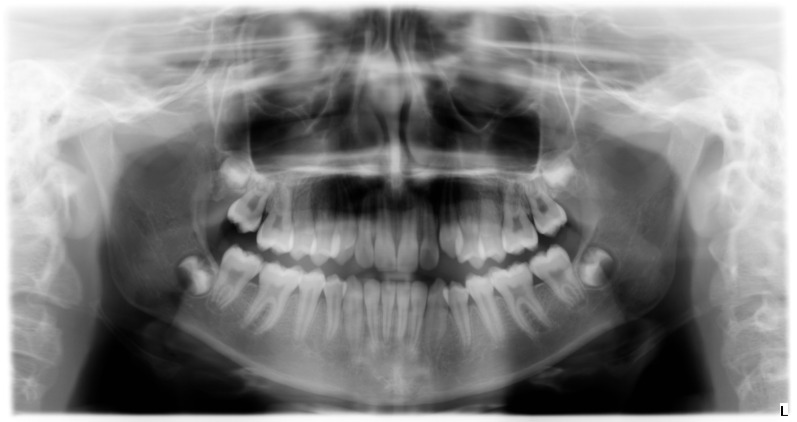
Radiographs of the patient at the screening. Orthopantomography X-ray after treatment and a 3-year follow-up period (12-year-old).
